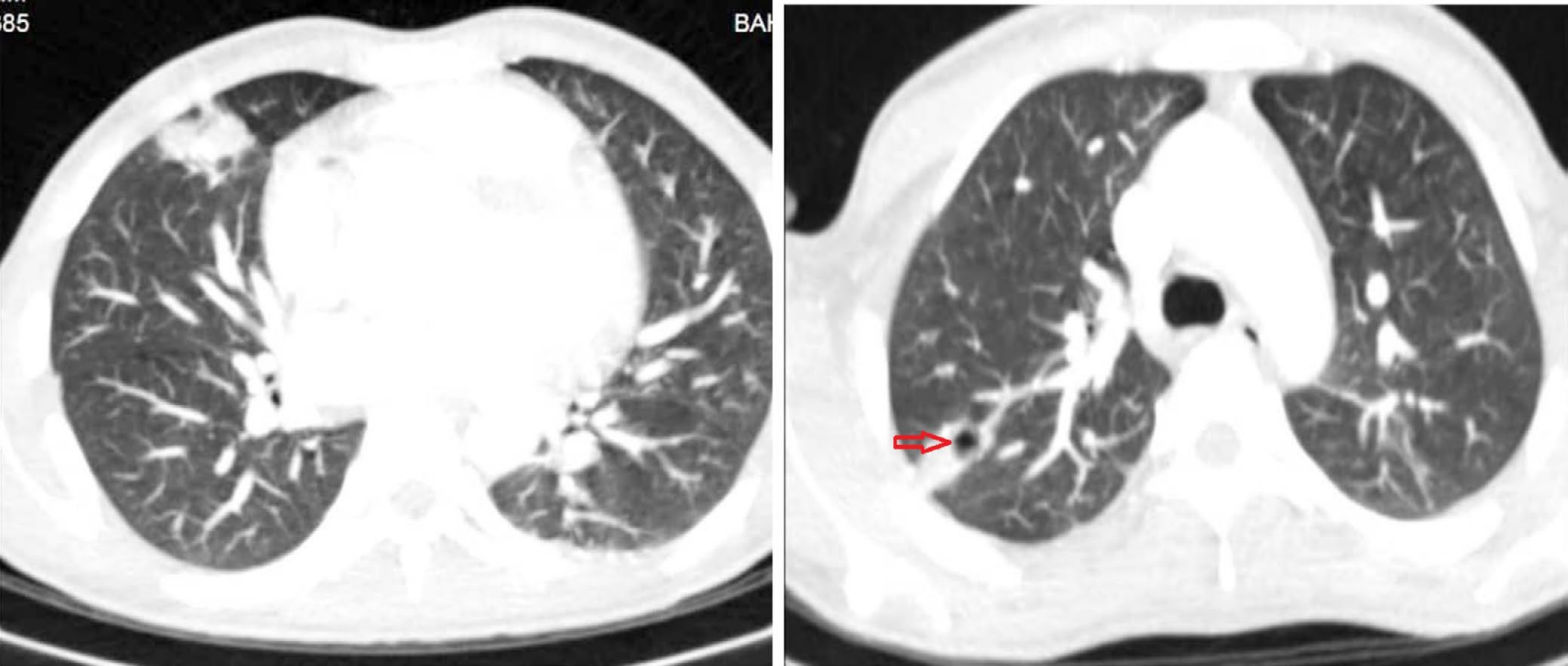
Chest CT of the patient that showed cardiomegaly with increased venous congestion (left) and a cavitary consolidation (red arrow) at upper lobe of right lung (right)
